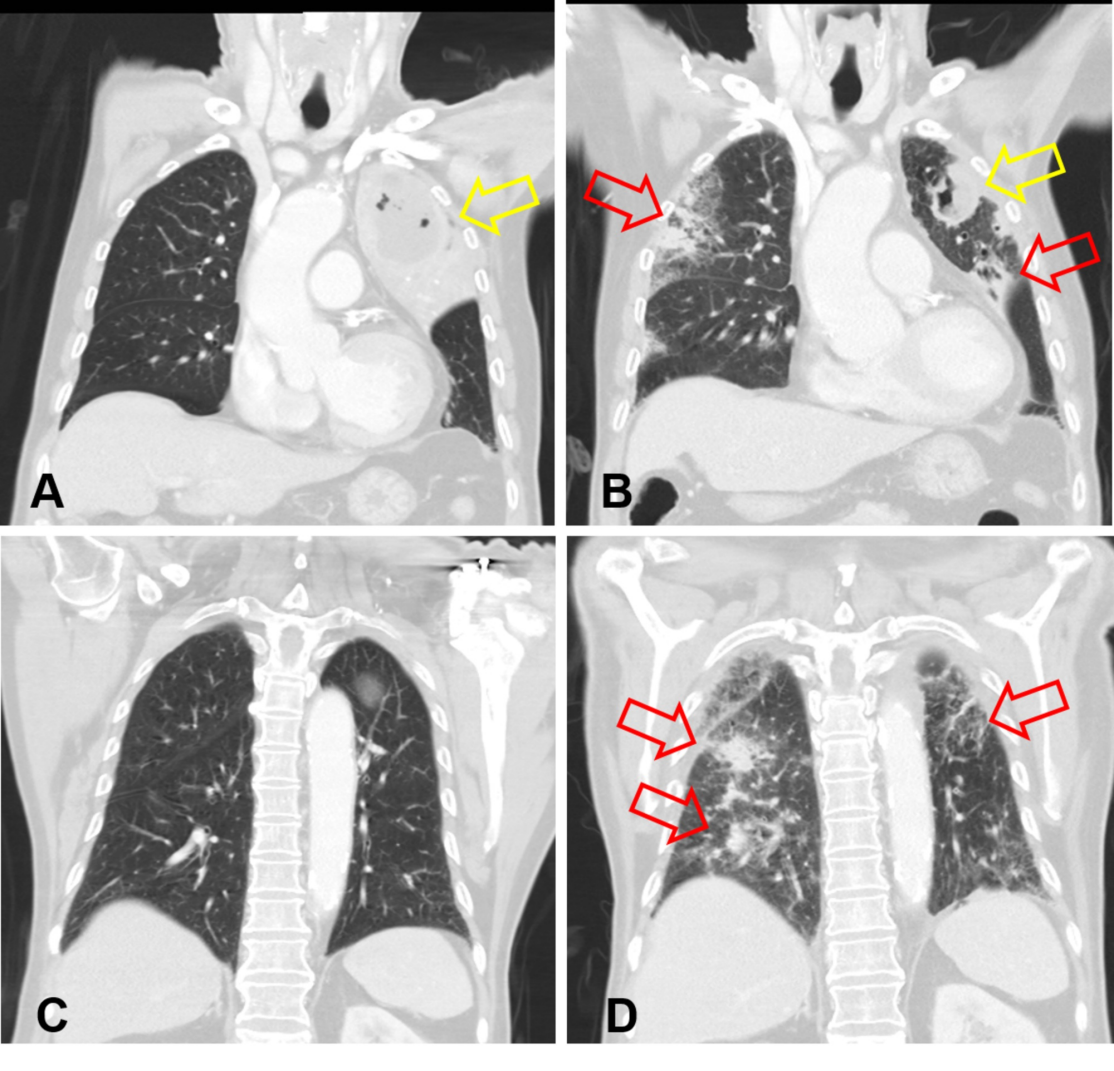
CT scan (lung window) disclosing development of pneumonitis (red arrows) along with tumor necrosis (yellow arrows) after therapy: A and C. February 21, 2019. B and D. June 12, 2019
